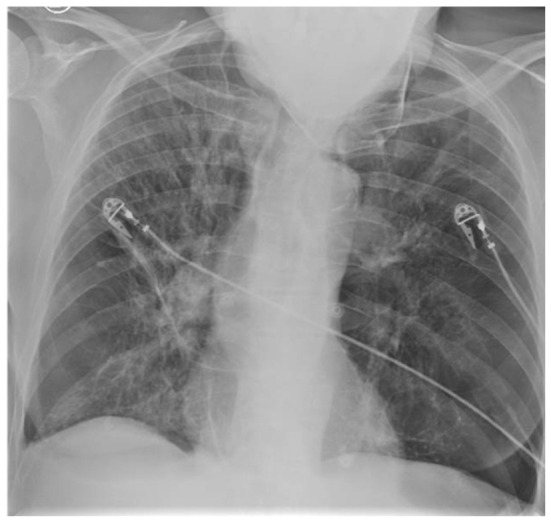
Chest radiograph obtained at presentation demonstrating hyperinflated lung fields with flattening of the diaphragms and attenuated peripheral vascular markings consistent with diffuse panlobular emphysema. A right basal consolidative infiltrate consistent with pneumonia is also present.
